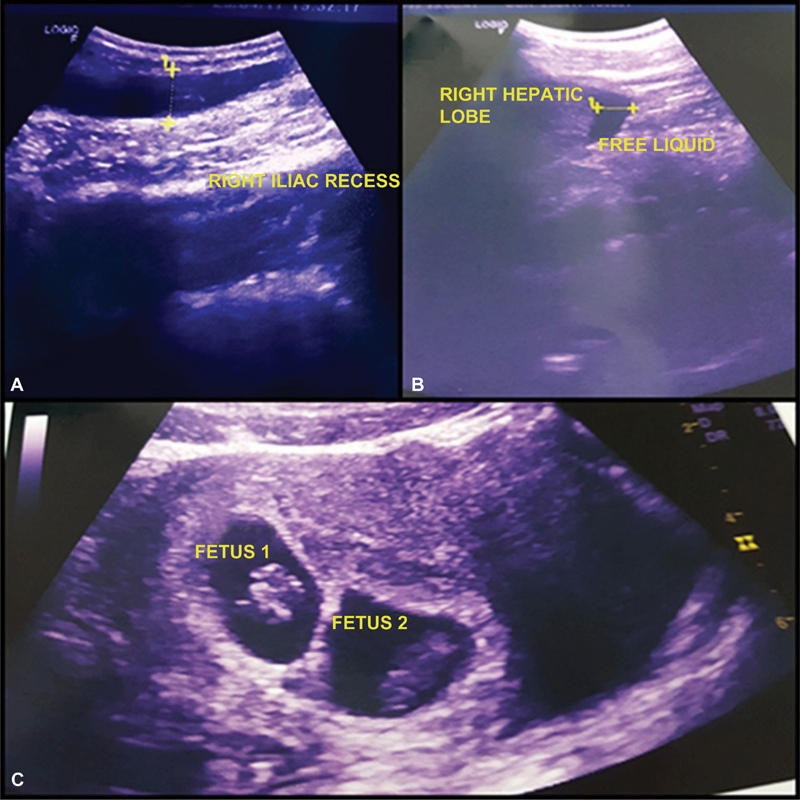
An ultrasonography had shown a 2 centimeters tubular structure in the right iliac fossa reported as acute appendicitis (A), presence of fluid in the perihepatic space (B), and 8-week viable intrauterine twin gestation (C).
